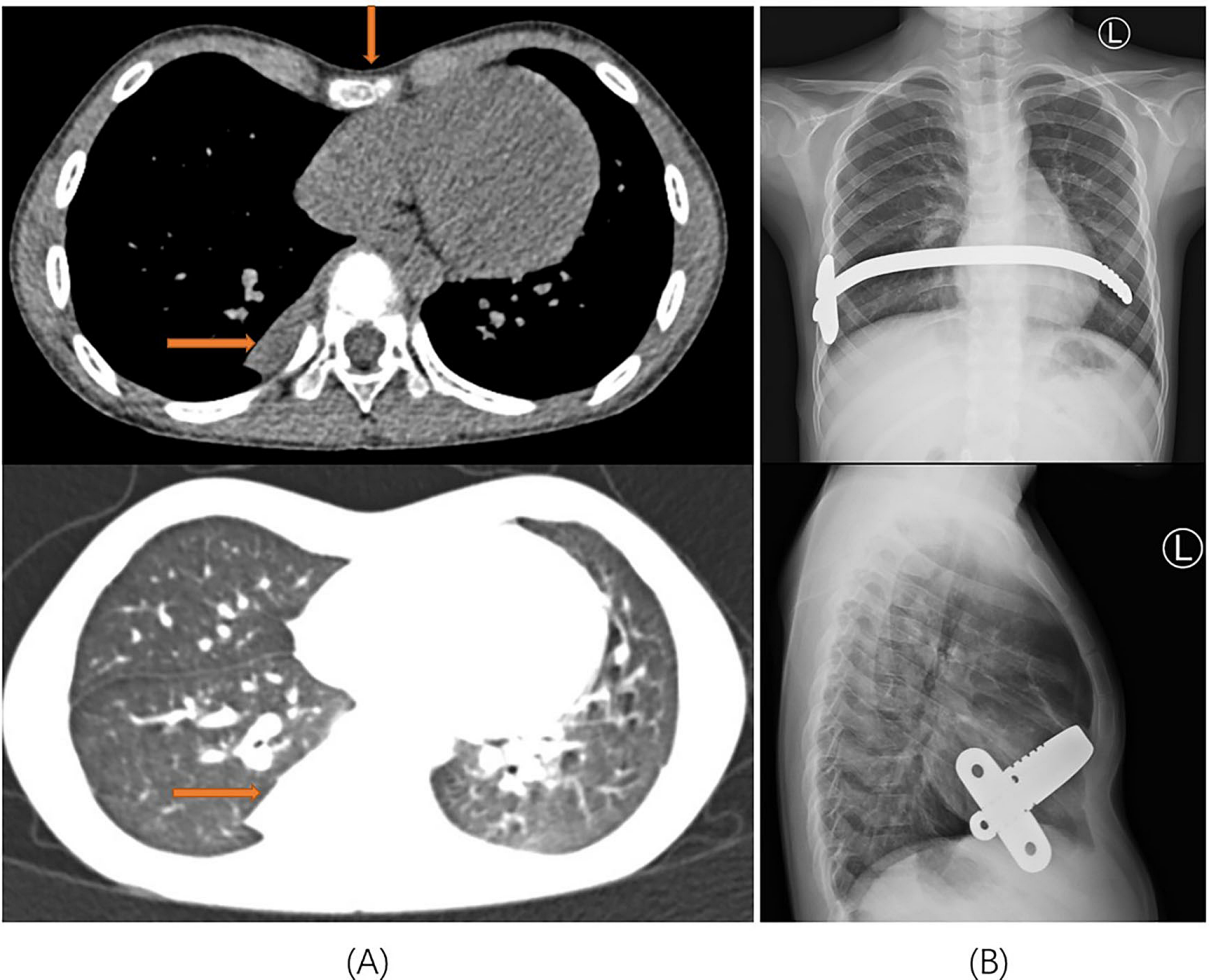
Preoperative chest CT and chest X‐ray 2 months after operation in patients with pulmonary sequestration complicated with pectus excavatum. (A) Preoperative chest CT tomography showing that the middle segment of the sternum and the corresponding bilateral costal cartilage are symmetrically depressed, with a range of approximately 13 × 14 cm, and the deepest depression was approximately 2.0 cm. A soft tissue density shadow can be observed in the lower lobe of the right lung, and pleural thickening can be observed between the two lung lobes. (B) Two months after surgery, the patient returned to the hospital for review of chest radiographs in the front and side positions. The orthopedic plate was well fixed, thoracic depression significantly improved, and the left lower lobe changed after the operation.
